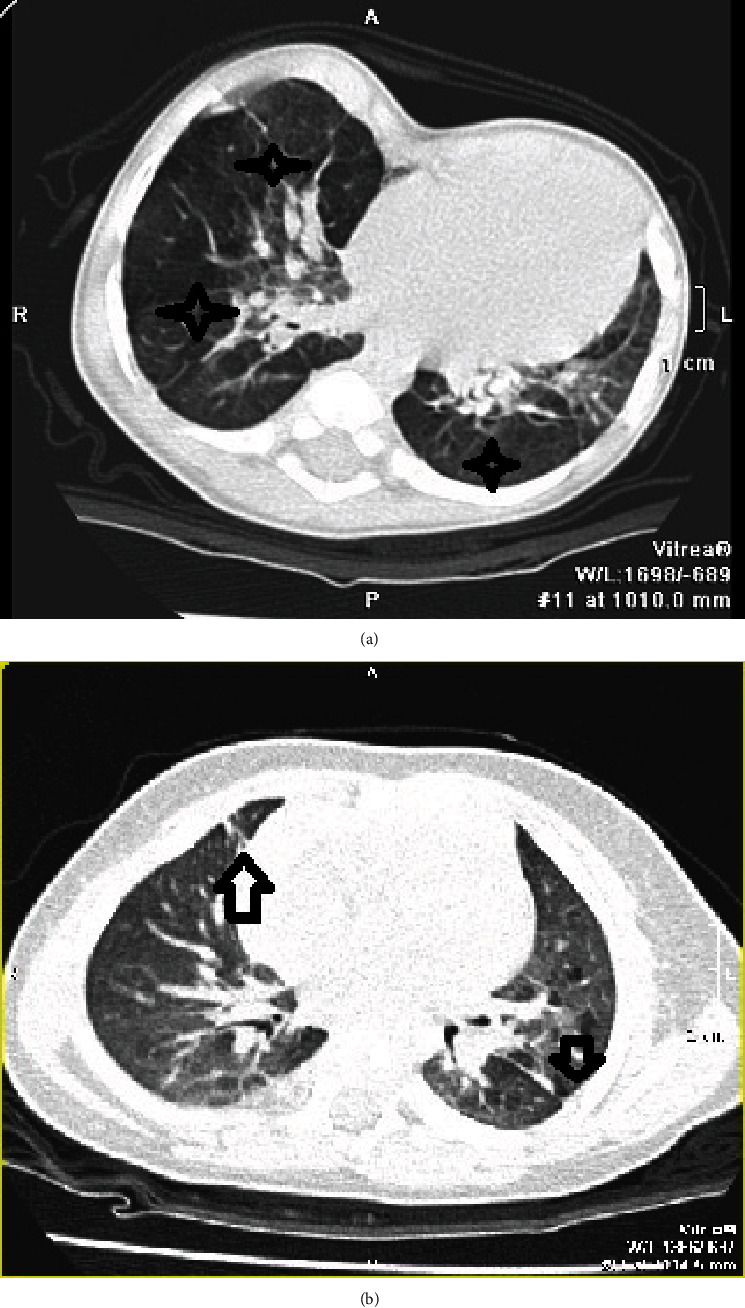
The most common abnormalities in HRCT scans. (a) Stars: the decreased pulmonary attenuation (mosaic perfusion). (b) Arrows: linear/triangular subpleural opacity.
